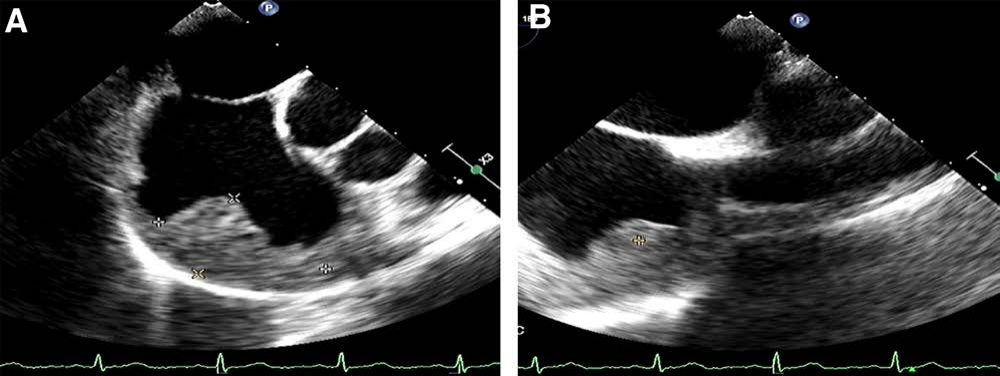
Transesophageal echocardiography of the heart. (A and B) A hypoechoic mass was detected in the right atrium near the opening of the superior vena cava, about 43 × 26 mm in size, with clear boundary, irregular shape, and heterogeneous internal echo.
